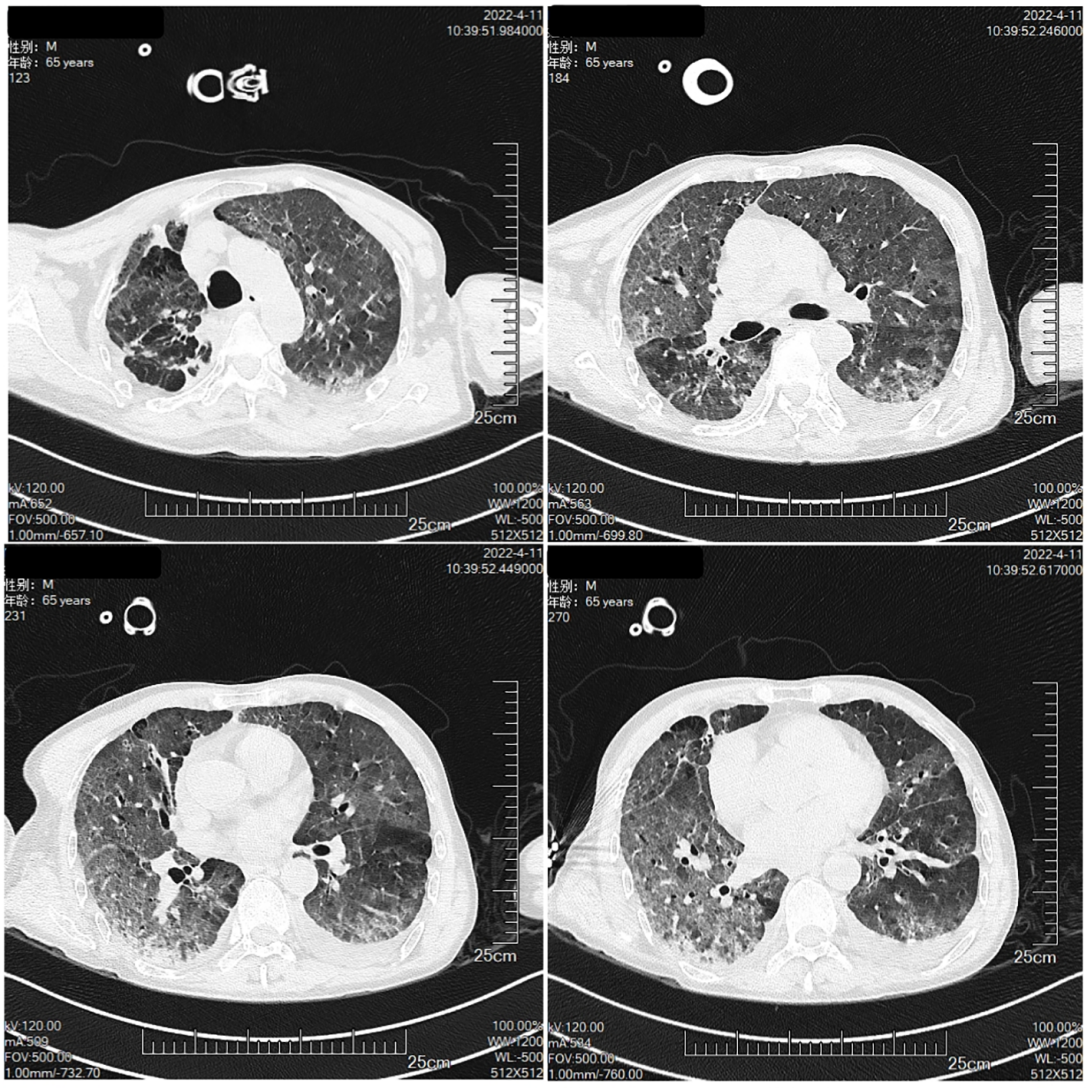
The Chest CT on April 11th.
